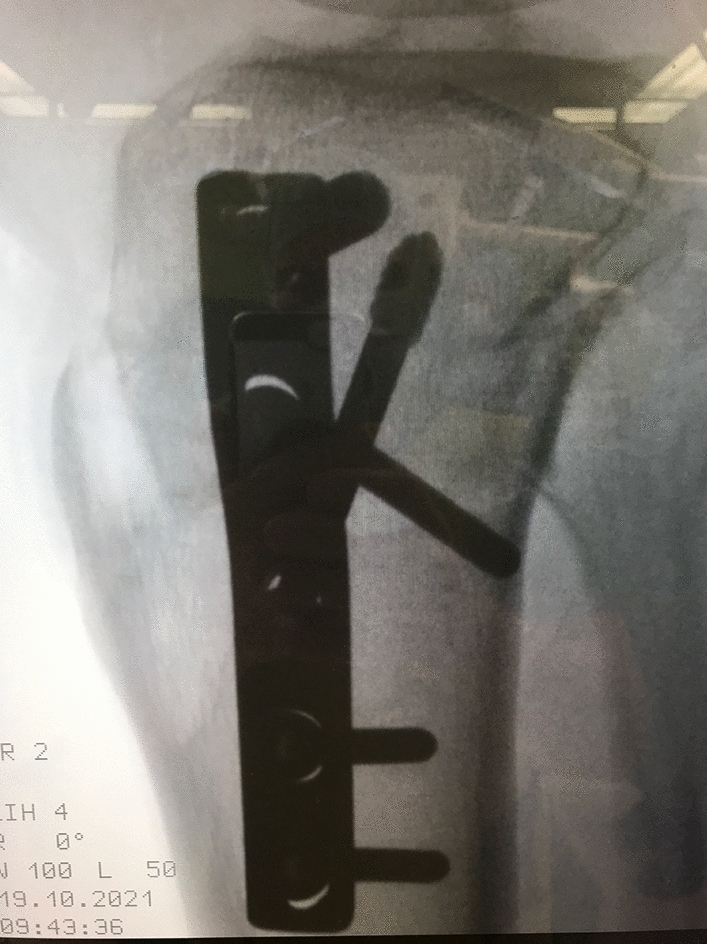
Intraoperative x-ray lateral view after the tibial internal torsional osteotomy as the first step of a double-level torsional osteotomy. The osteotomy was performed and osteosynthesis was done with a 5-hole DCP plate tibial. In the lateral view the infratuberositary level of the osteotomy leading to a supratuberositary level at the ventral aspect can be seen
